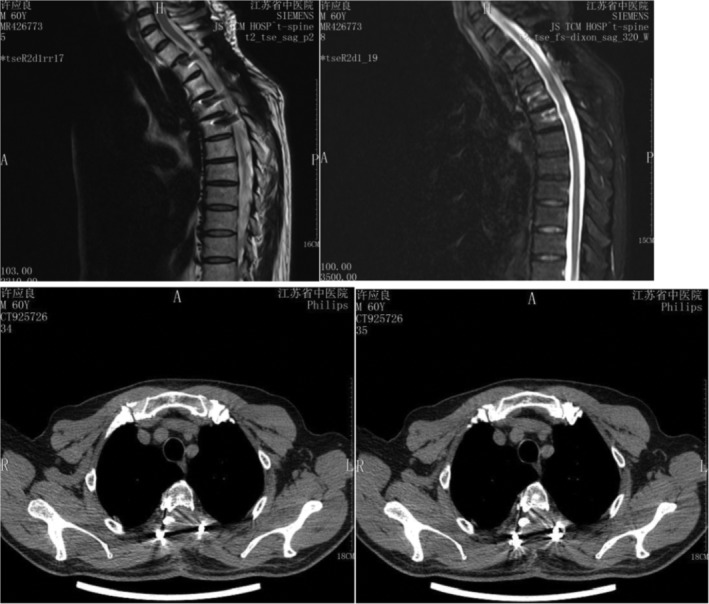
MRI and CT scan before SBRT precision radiotherapy.
